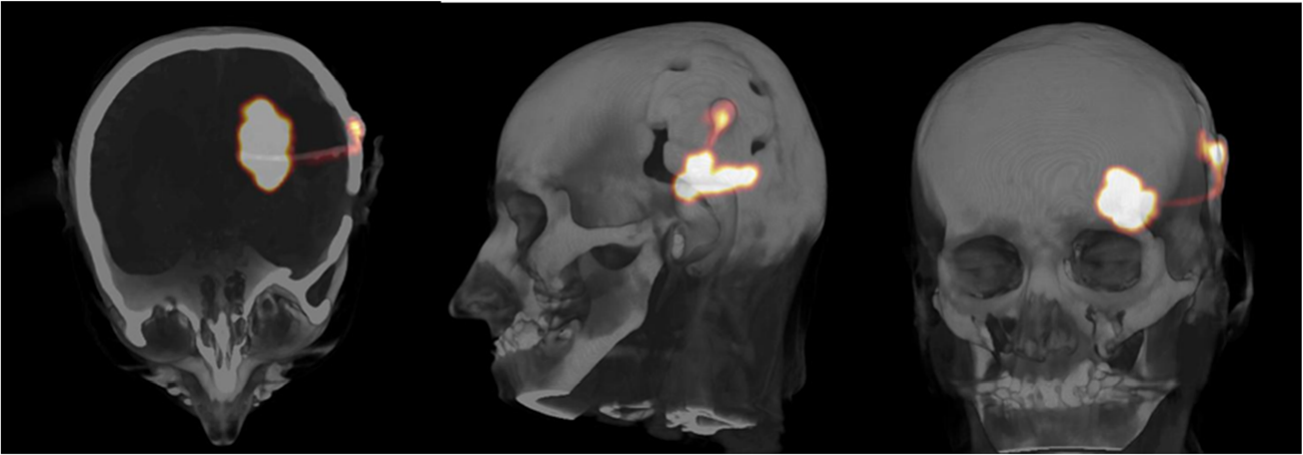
PET/CT after local co-injection of 10 MBq ^68^Ga-DOTA-SP with a therapeutic dose of ^213^Bi-DOTA-SP into the resection cavity of a glioblastoma in patient 2. Most of the activity is concentrated within the lesion, with little activity remaining in the capsule and within the catheter
